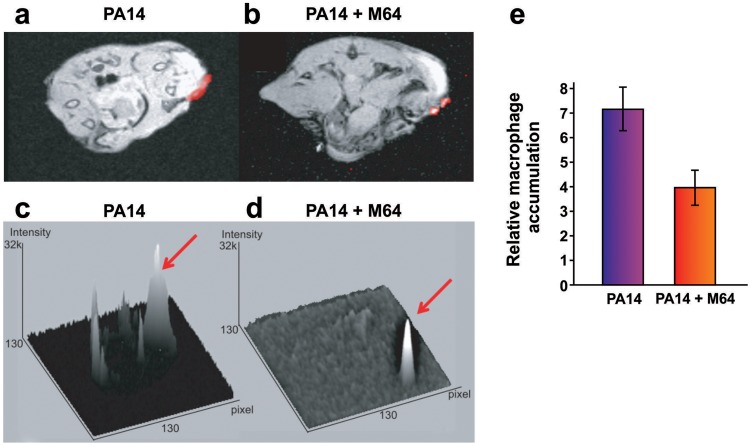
Magnetic resonance imaging of M64 inhibition of macrophage recruitment at a burn and infection site. a–e.
